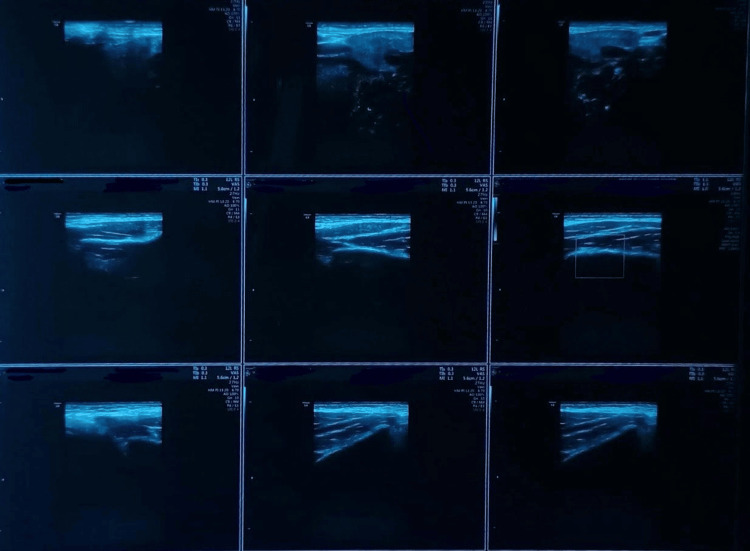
Colour Doppler study of left angle of mandible
